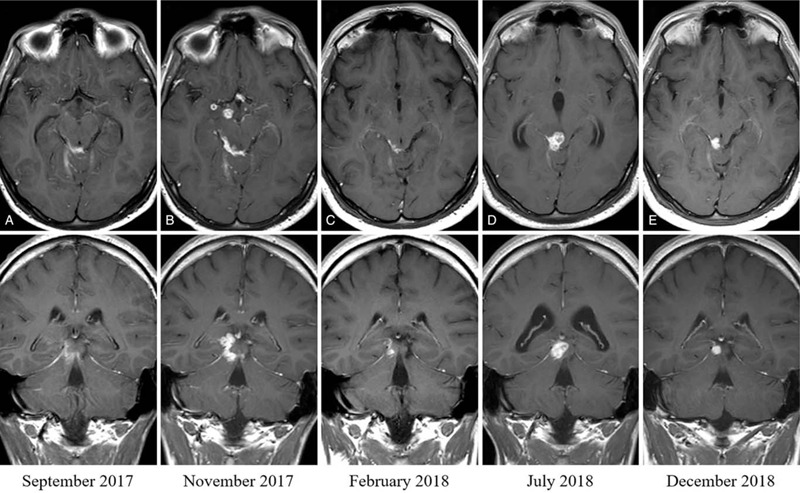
Axial and coronal contrast-enhanced T1-weighted magnetic resonance images of case 2. At diagnosis (September 2017): extraparenchymal enhancing lesion on the right ambient cistern infiltrating the right superior colliculus (A). Six weeks later (November 2017): appearance of new tuberculomas in midbrain tegmentum, suprasellar cisterns and right temporal uncus (B). After 3 weeks of a three-dose course of infliximab (February 2018): Remarkable reduction of tuberculomas and leptomeningeal enhancement (C). On the eleventh month of tuberculosis treatment (July 2018): obstructive hydrocephalus secondary to mass effect over Silvius aqueduct (D). And 3 months after discontinuation of tuberculosis treatment (December 2018): Mild leptomeningeal enhancement, with resolution of the mass effect (E).
